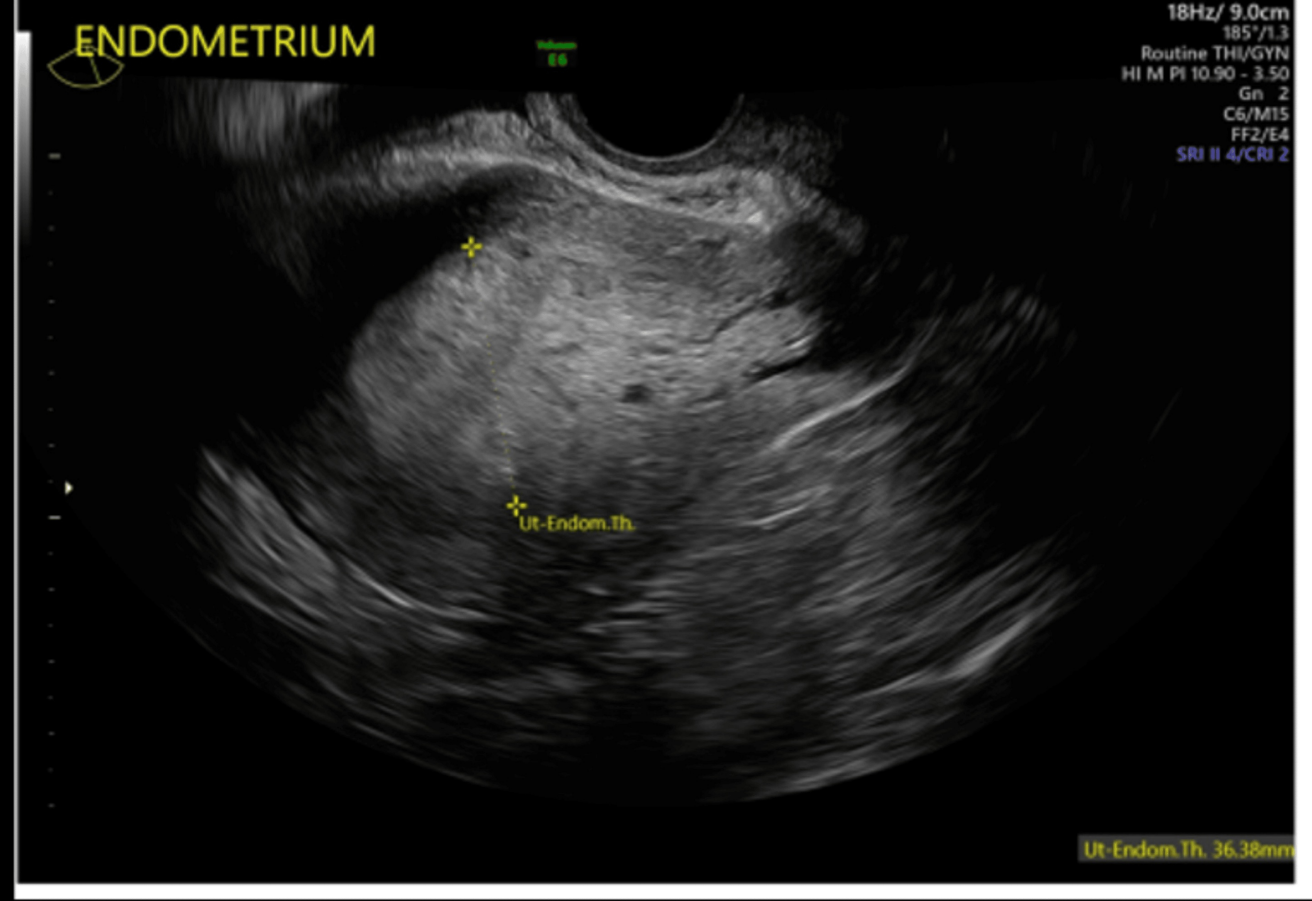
Ultrasound image of the endometrium, demonstrating an endometrial stripe of 36.88 mm.
